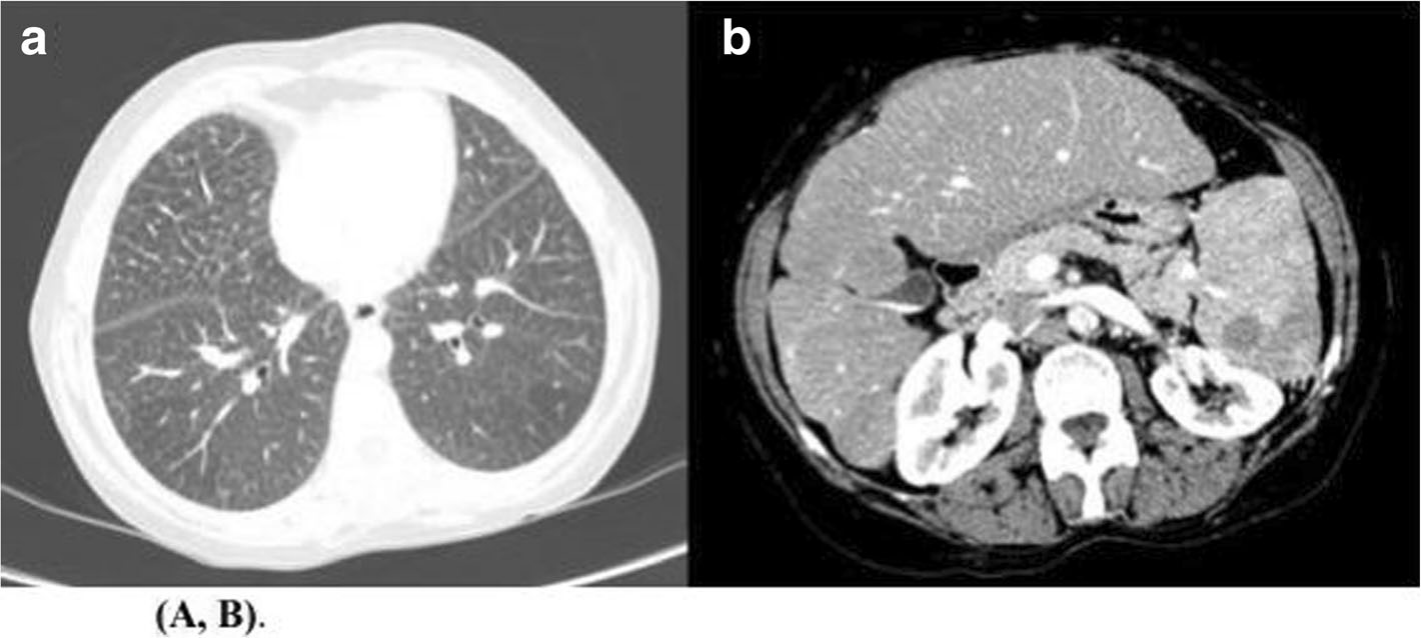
Computerized tomography (CT). a. Crazy-paven pattern of the lungs with calcified granulomas. b. Hepatic cirrhosis with hypodense nodes on the left hepatic lobe, signs of portal hypertension and splenic nodes
